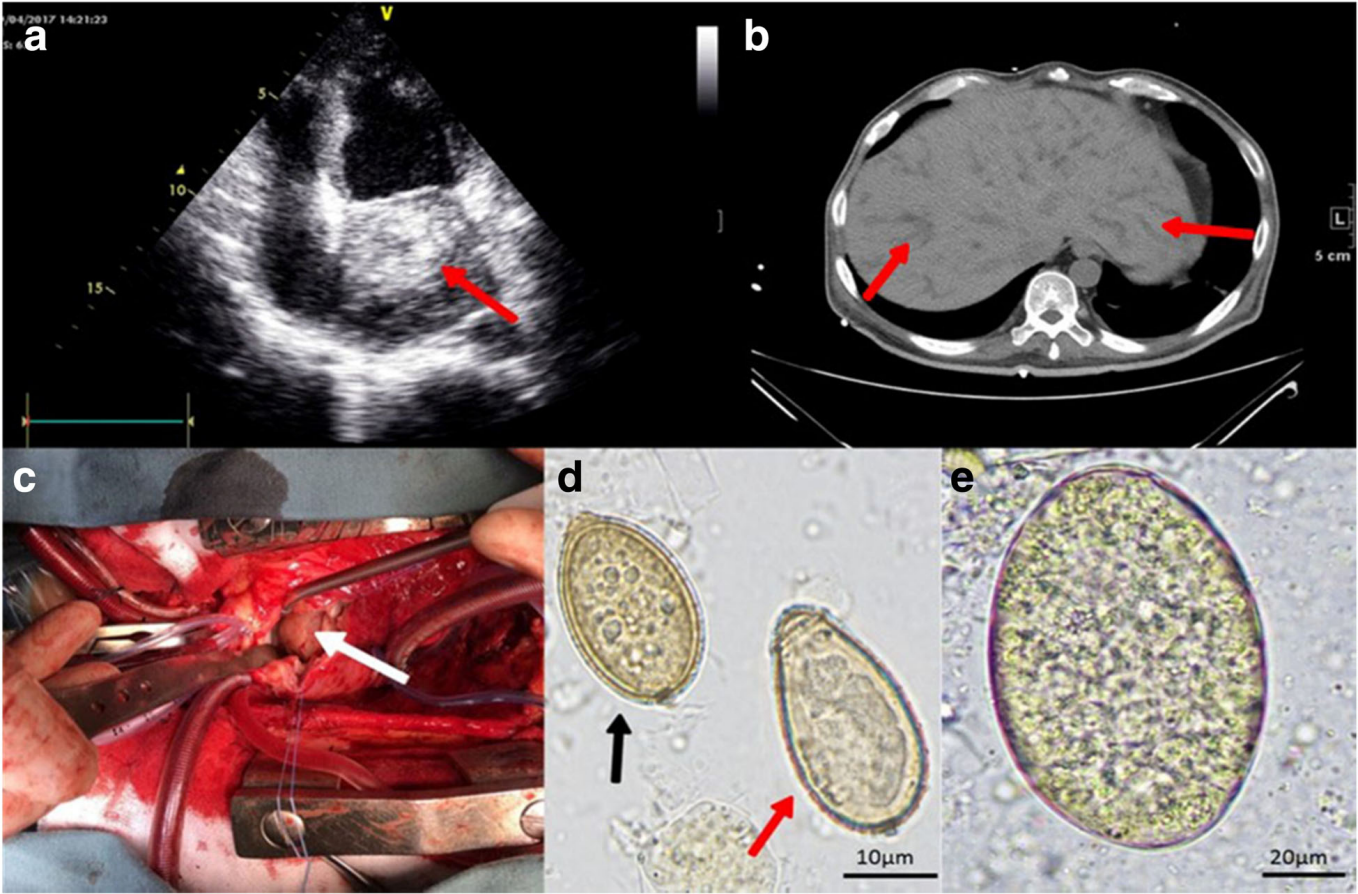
Imaging, surgical and pathogenic findings in the patient. (a) A space-occupying lesion (red arrow) was observed in the left atrium measured 60 × 54 mm by cardiac ultrasonography. (b) Abdominal CT scan showed extensive intrahepatic bile duct dilatation (red arrow). (c) The round cardiac mass (white arrow) in the left atrium was exposed in the surgery, which was an endocardial hematoma close to the posterior mitral valve. (d) The egg of *C. sinensis* (red arrow) was firstly identified in the stool samples, and later a smaller egg (black arrow) consistent with that of heterophyid trematodes was distinguished from *C. sinensis*. (e) Larger-sized eggs in the stool samples were consistent with those of the Echinostomatidae family
